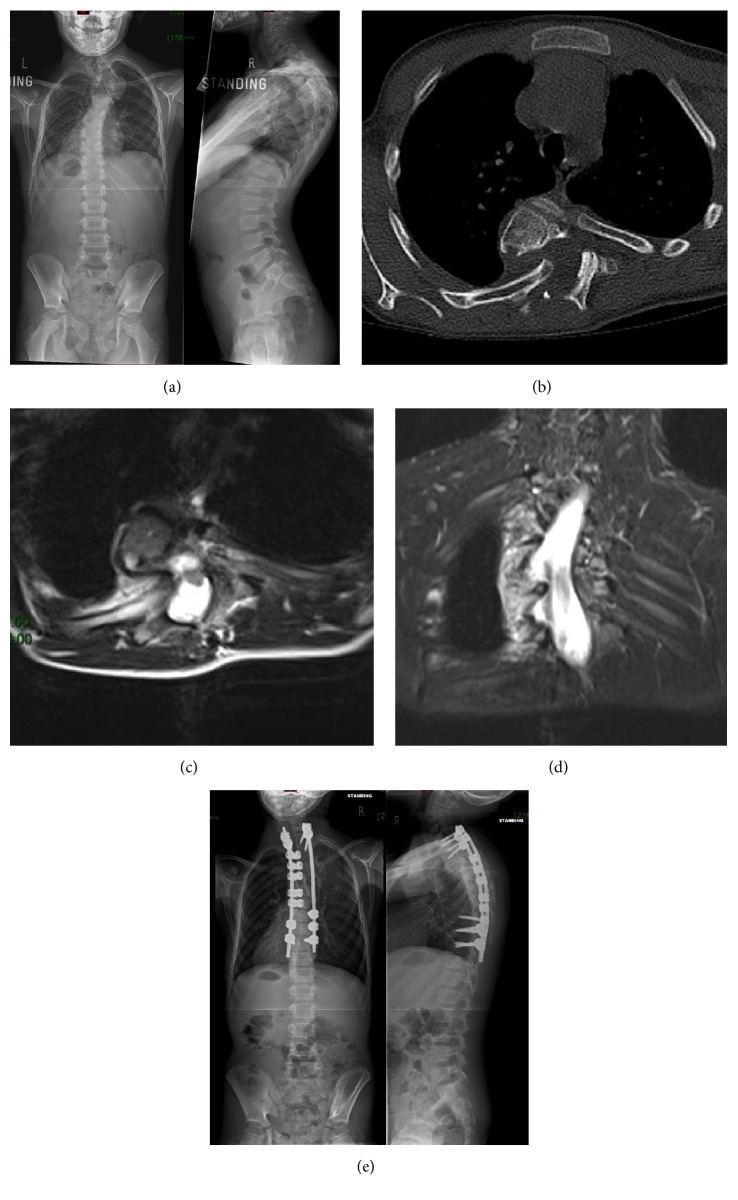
Preoperative whole spine AP and lateral X-ray (a). Preoperative CT scan (b) demonstrating the rib head intracanal dislocation. Preoperative sagittal T2 MRI axial (c) and coronal (d) views demonstrating the rib head in close proximity to the cord but without impingement. Postoperative whole spine AP and lateral X-rays (e).
